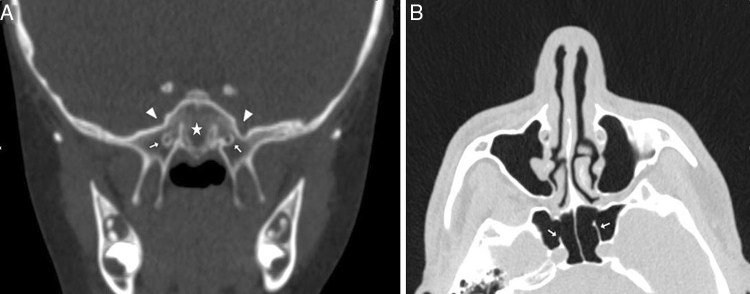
(A) Coronal CT section showing the conchal type sphenoid pneumatization pattern (star); (B) Axial CT section showing the intrasphenoid septum which deviated and attached on the carotid prominence (ICAS) (thick arrow).
